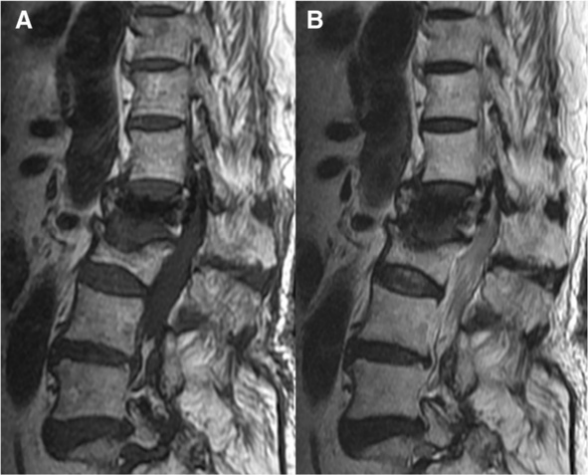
Sagittal MRI images. Sagittal T1-weighted MRI (A) and sagittal T2-weighted MRI (B) revealed bone cement leakage into the spinal canal with neural element compression.
